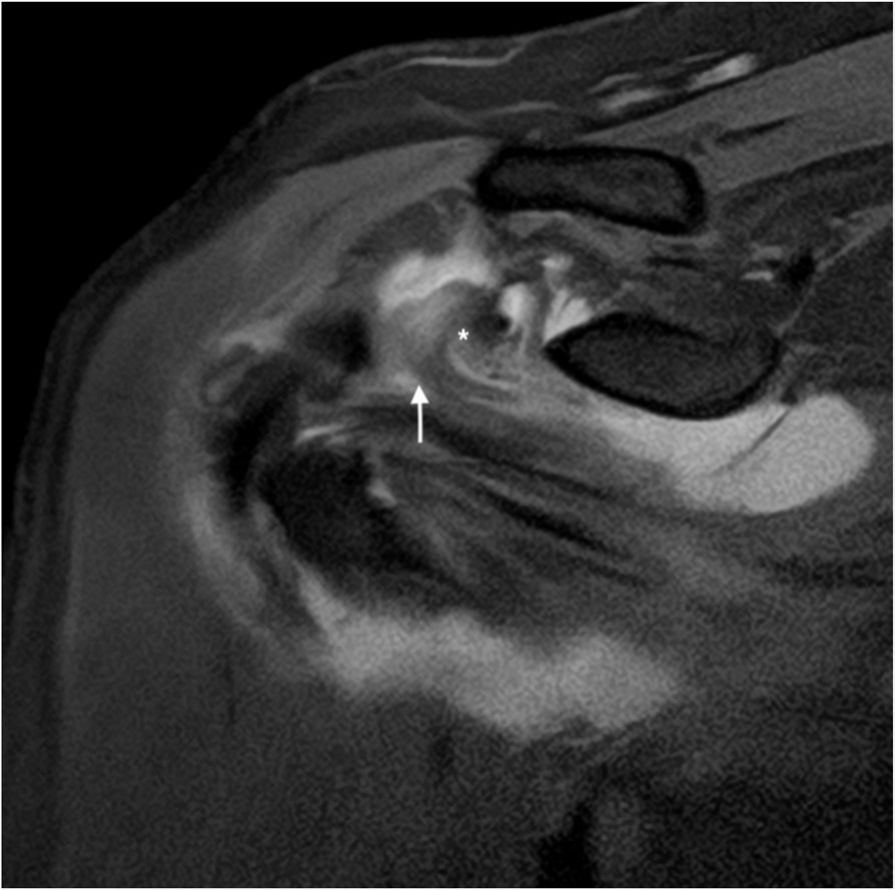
Bridging sign. Oblique coronal fat-suppressed PD-weighted MRI showing the torn cranial portion of the SSC tendon with superomedial displacement (arrow) due to retraction of the coracohumeral and superior glenohumeral ligament complex (asterisk)
